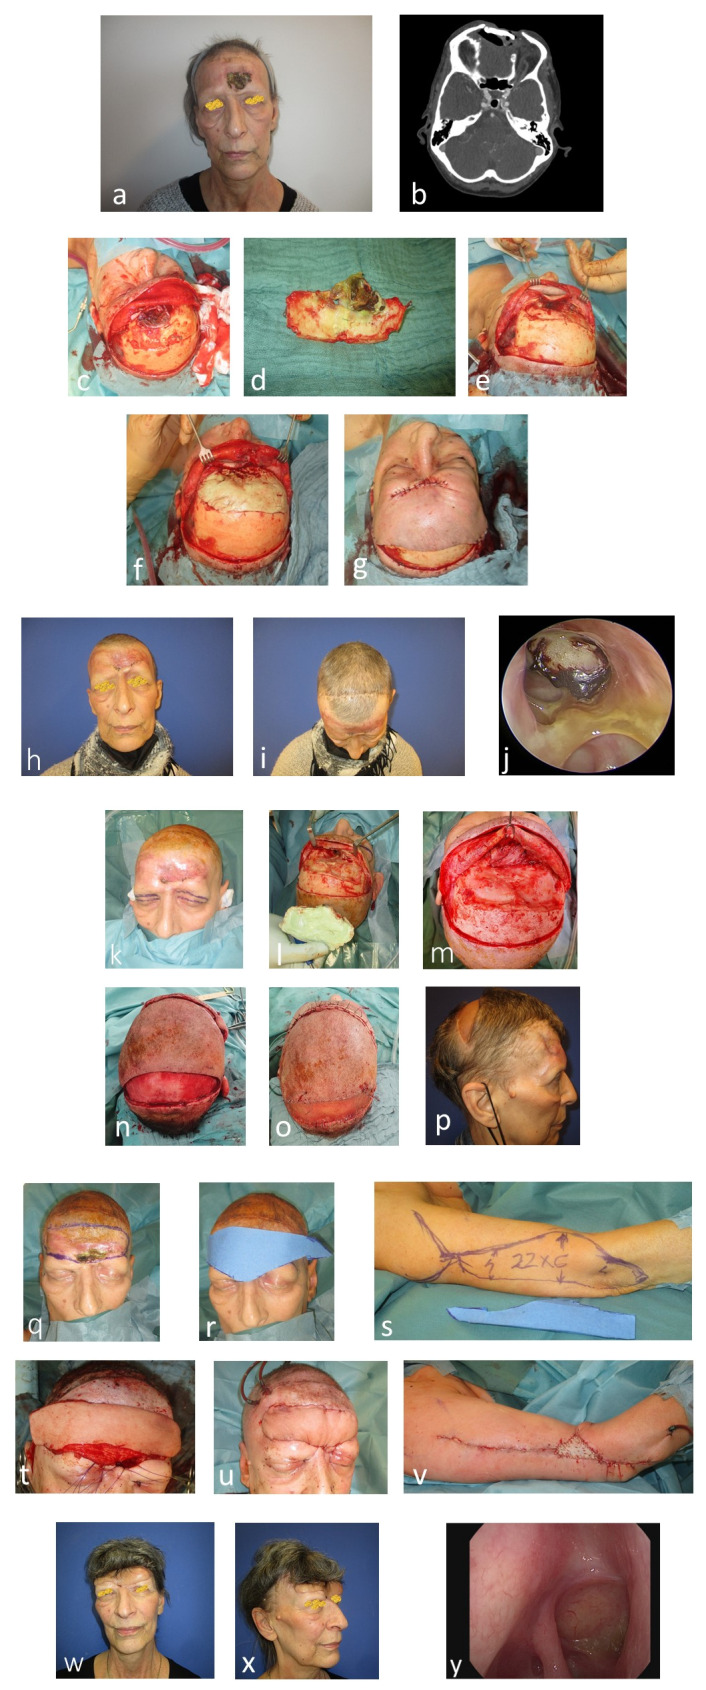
Case 3: 69-year-old female with a frontal soft tissue and bone defect after resection of a high-grade squamous cell carcinoma of the frontal sinus and filling with PMMA spacer a: Frontal soft tissue and bone defect. b: CT scan. c: Intraoperative finding. d: Resected necrotic frontal bone together with the PMMA spacer. e: Communication with the nasal cavity. f: Formed PMMA spacer with Vancomycin. g: Wound closure with visor flap and parietal undermining. h: Postoperative anterior view. i: Postoperative parietal view. j: Endoscopic view of the PMMA spacer. k: Second surgery. Planning for bilateral upper lid flaps to close the nasal cavity. l: Removal of PMMA spacer. m: Transplanted bilateral upper lid flaps. n: Visor flap. o: Split thickness skin grafting of the donor site. p: Postoperative side view. q: Skin necrosis frontal. r: Template of the planned flap with the removal of the whole frontal aesthetic unit. s: Lateral arm flap before harvesting. t: Transplanted flap; the lower part was deepithelized and turned over to close the nasal cavity. u: Sutured flap; no spacer was used at this surgery. v: Donor site. w: Postoperative anterior view six months postoperative. x: Postoperative side view six months postoperative. y: Endoscopic view with closed communication to the frontal region.
